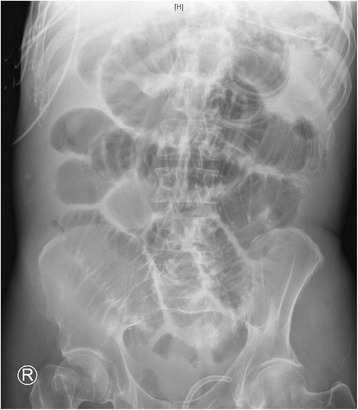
Abdominal X-ray showing diffuse small bowel dilation
